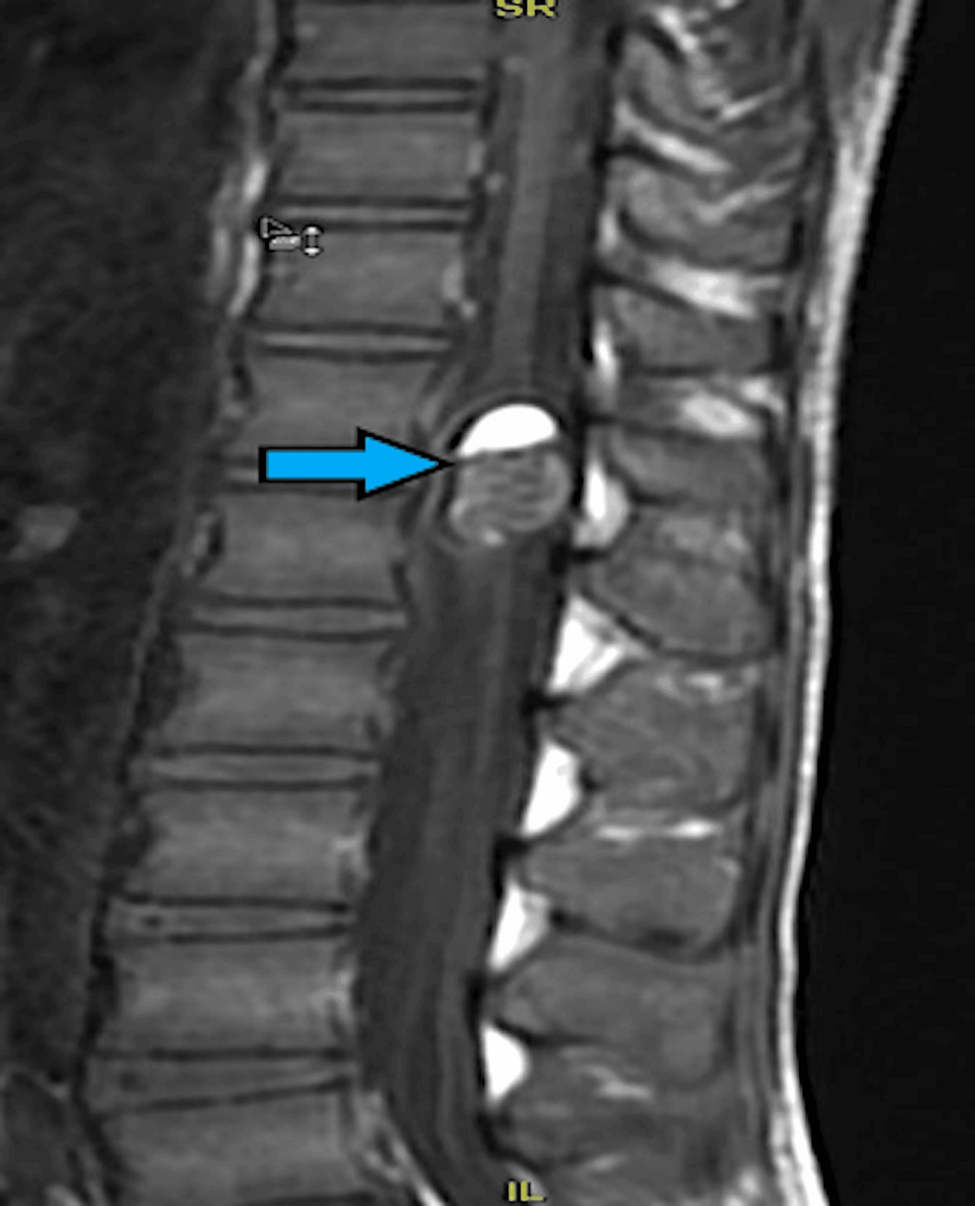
T1-weighted MRI depicts a well-defined oval intramedullary lesion within the spinal cord at D12-L1 level causing expansion (size: 1.4 cm anteroposterior and 1.85 cm transverse) more towards the left side of the cord with areas of T1 hyperintensity (blue arrow).
